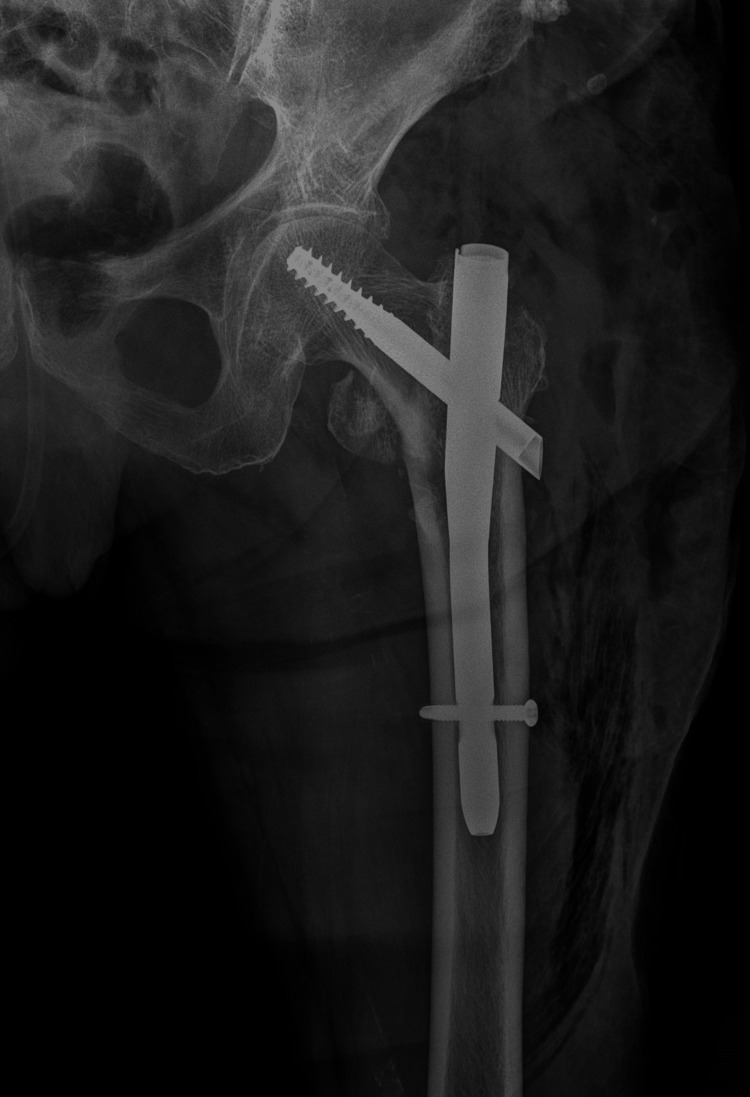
Postoperative radiograph of the left hip showing fracture fixation with a cephalomedullary nail
